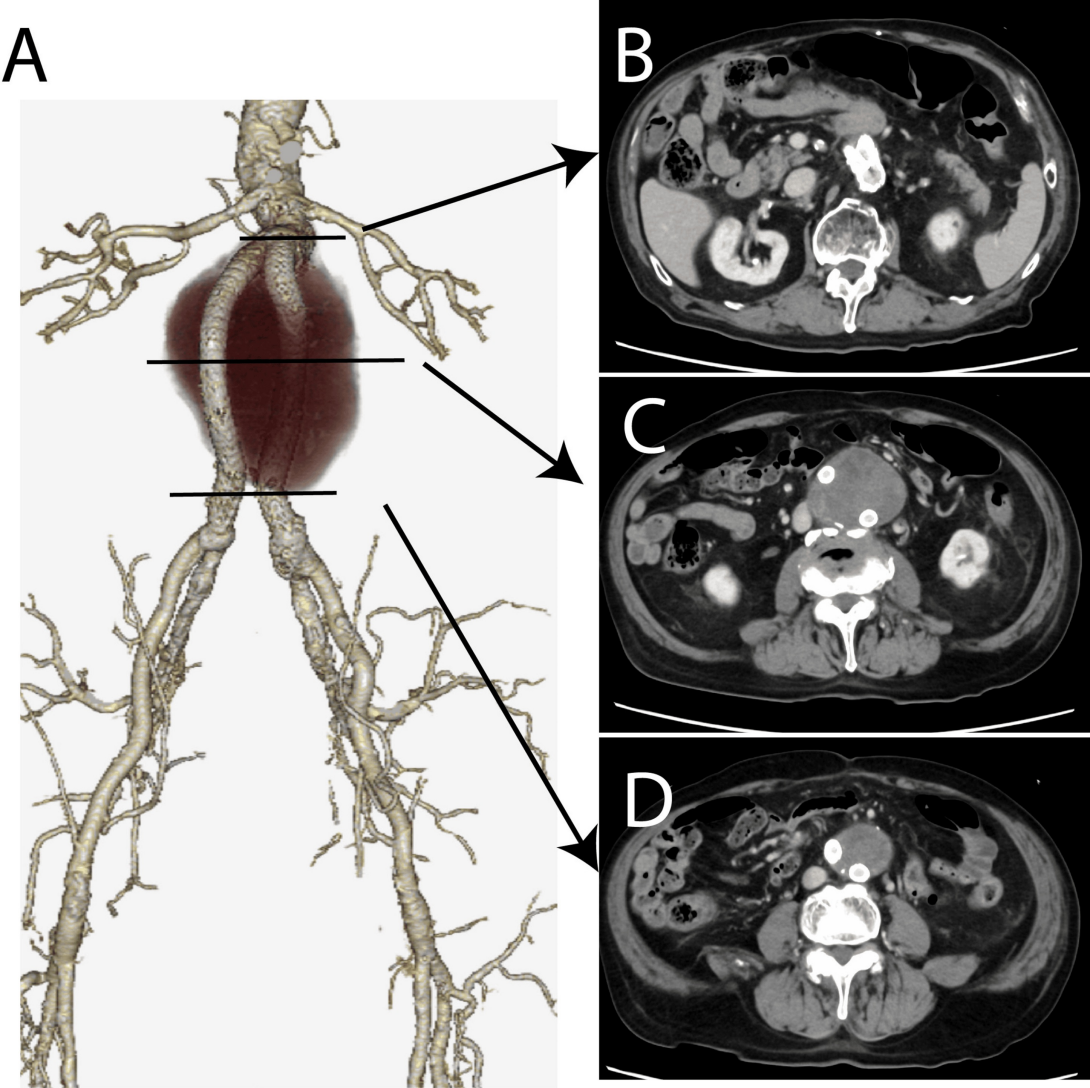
Contrast computed tomography image taken after endovascular therapy. (A) Volume rendering image. (B) No leak from an artificial blood vessel is visible at the proximal site. (C) Middle portion. (D) Distal site.
